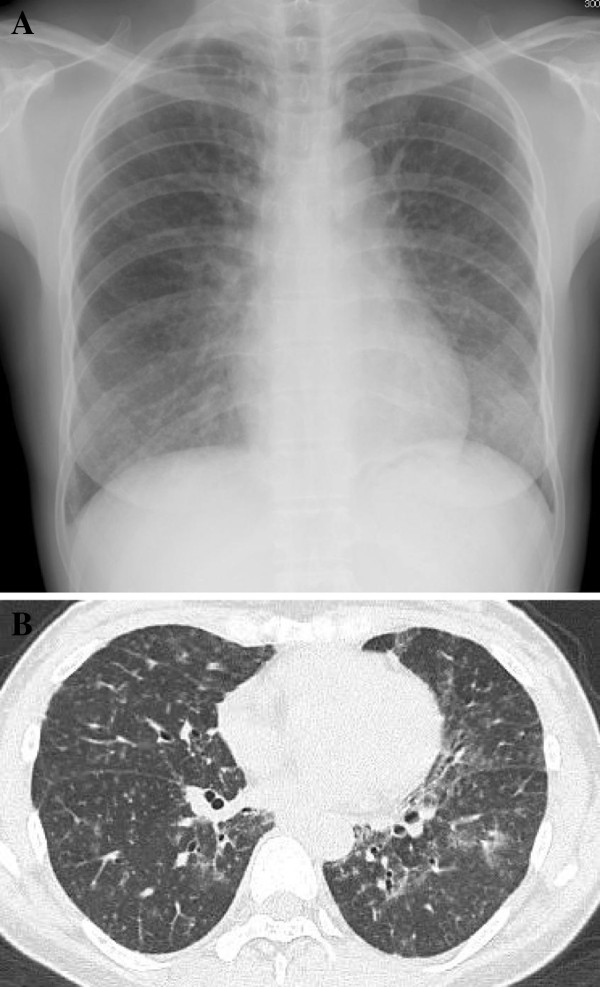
Radiological findings. A chest X-ray on admission, showing fine and diffuse nodular shadows in both lung fields (A). An initial CT scan of the chest demonstrated randomly-distributed micronodules and patchy ground-glass opacities (B).
